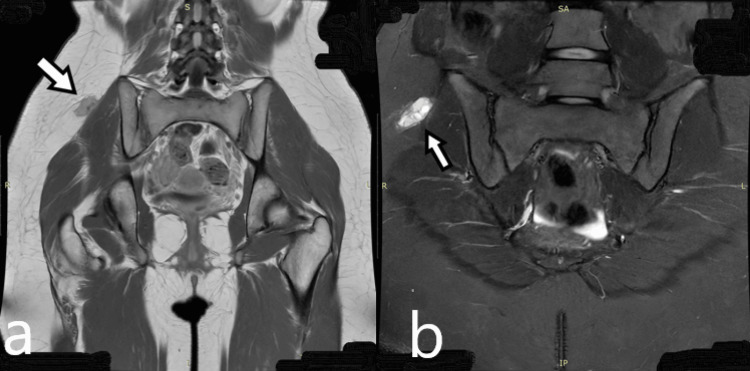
MRI images after two months (a) Coronal contrast-enhanced T1W MRI after two months from the initial MRI shows shrinking of the lesion, measuring 2.1 × 1 cm. (b) Coronal FSE STIR MRI obtained two months after the initial MRI shows reduced edema in the gluteal and paravertebral muscles. FSE, fast spin echo
